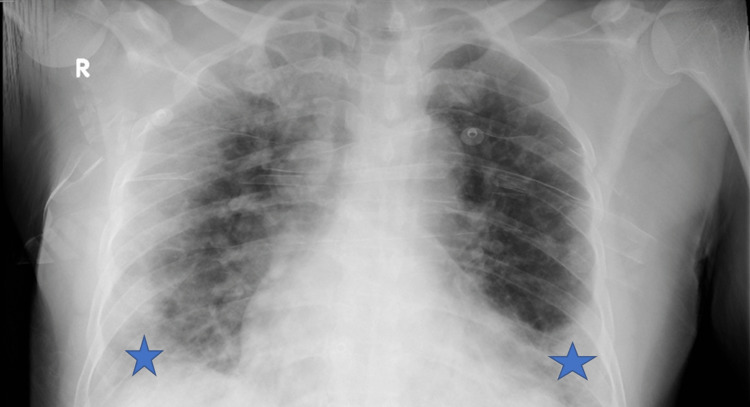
Chest X-ray shows COVID-19 related infiltrates in both lung fields and bilateral pleural effusion (Stars)
